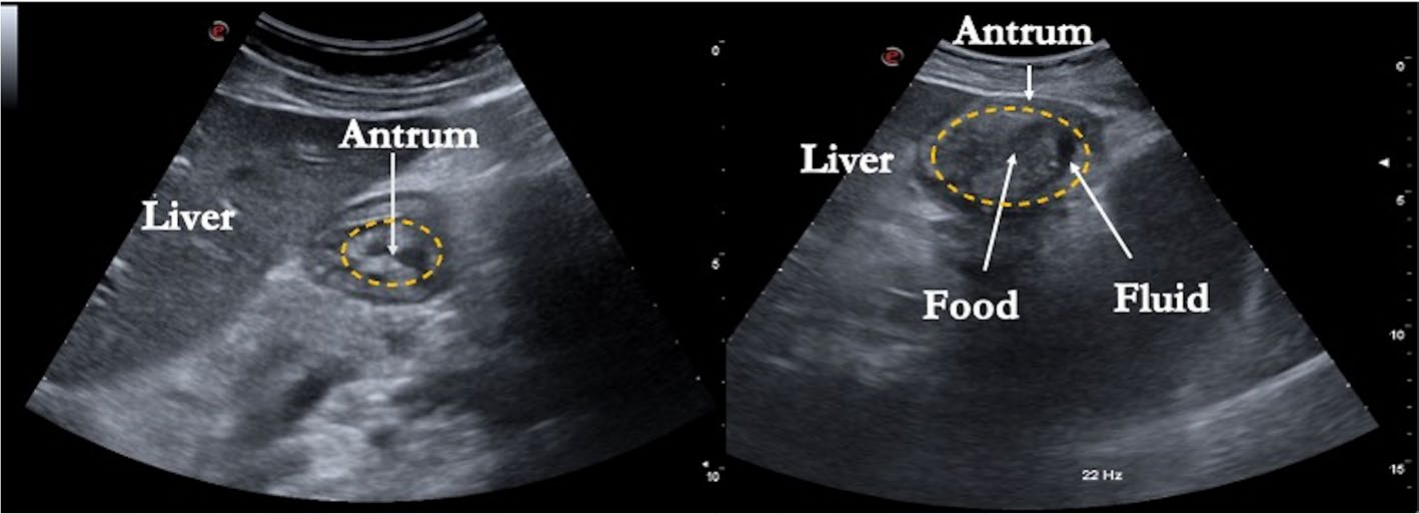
Gastric ultrasound evaluation
